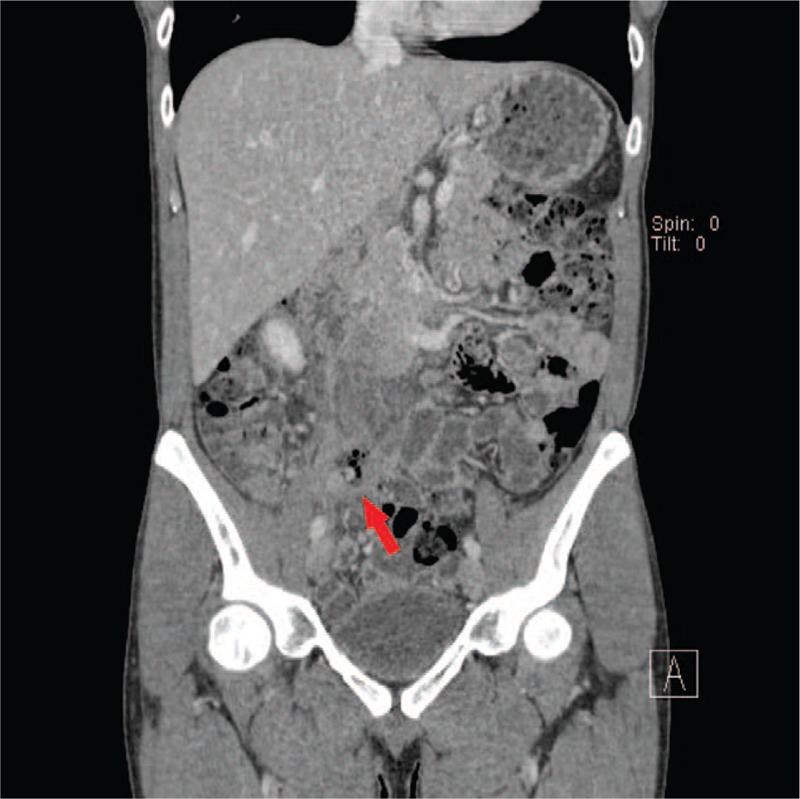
Contrast-enhanced abdominal and pelvic computed tomography in case 4. A perforation of the tip of stump appendix (arrow).
